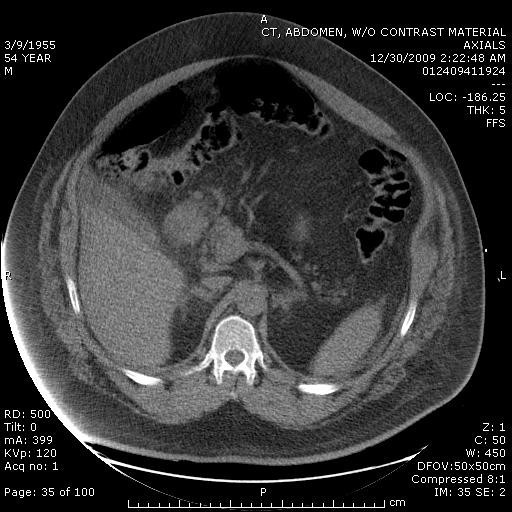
Non-contrast CT of abdomen revealing intra-abdominal free air and perforated viscus.
